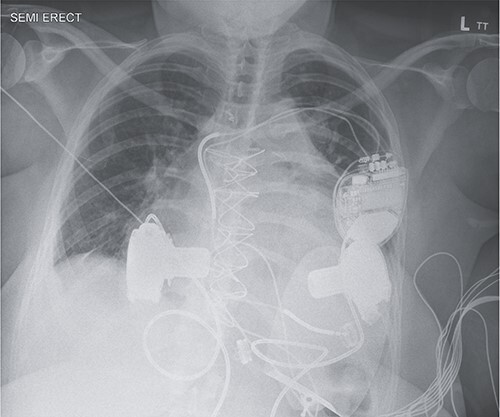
Anterior–posterior chest X-ray following BiVAD implantation.
